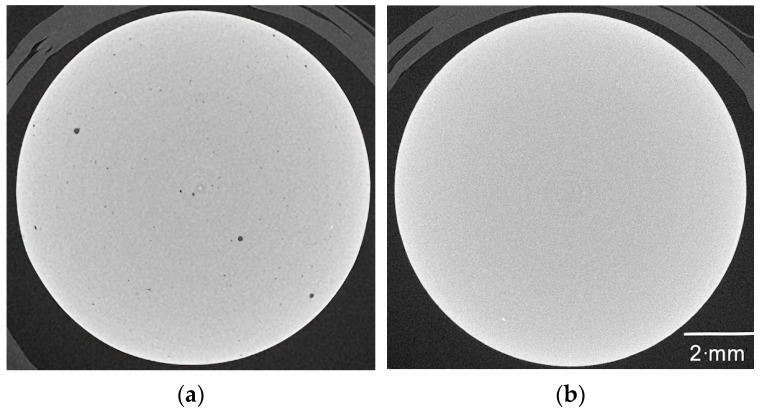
CT scans of sintered glass samples when applying feedstock 5 for replication: (a) only thermal debinding; (b) combination of liquid pre-debinding and thermal debinding.
